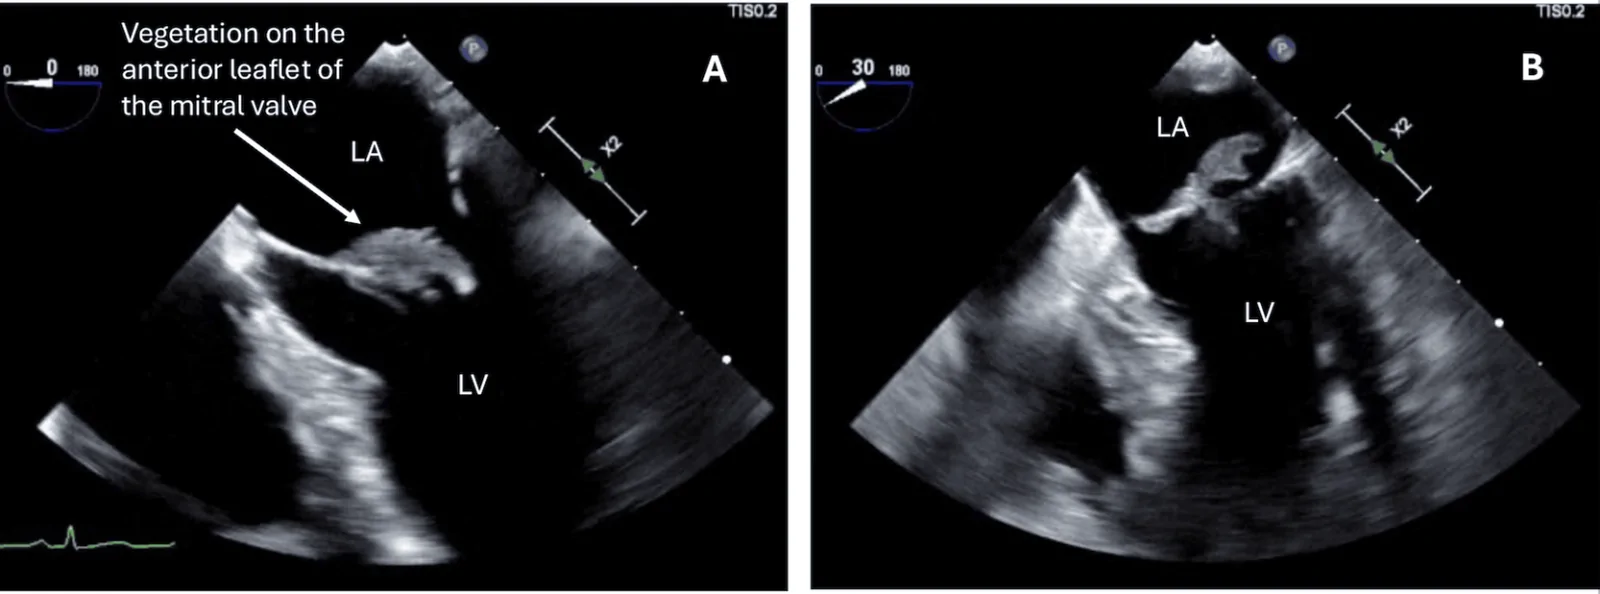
TEE in the mid-esophageal long-axis view with a large, mobile vegetation on the atrial aspect of the anterior leaflet of the mitral valve (A) during diastole and (B) systole, confirming the diagnosis of infective endocarditis TEE - transesophageal echocardiogram; LA - left atrium; LV - left ventricle
